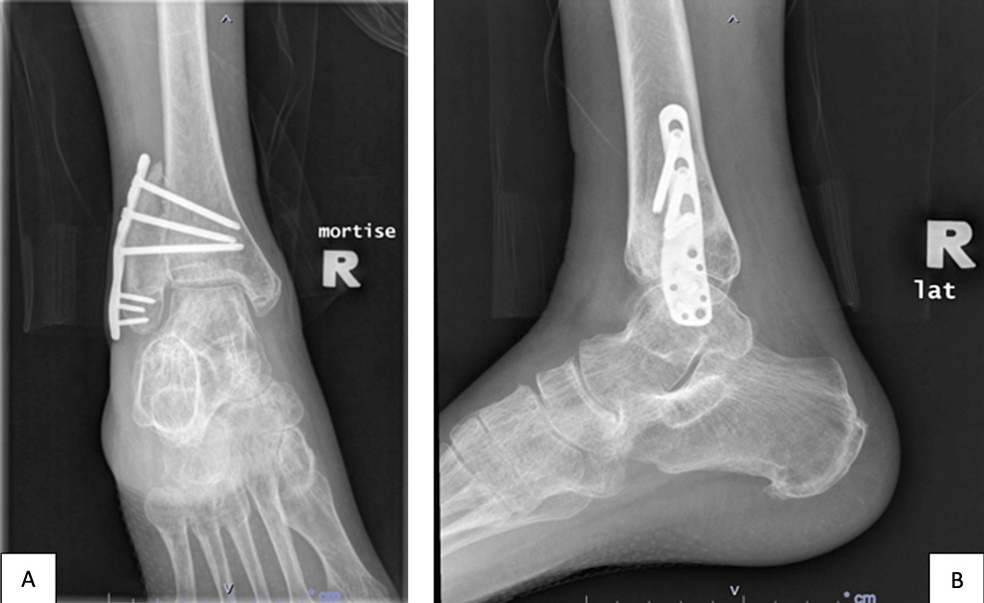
Two-year post-operative radiograph of the right ankle showing the fusion of the graft at the distal tibiofibular joint in mortise (A) and lateral (B) views
